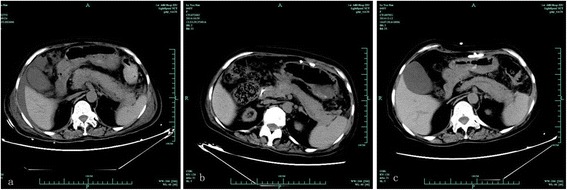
image of CT scan during the the whole course of AP. a Twentie-five days post transplantation, the CT scan of abdomen showed pancreatic swelling, peripheral exudate effusion, considered acute pancreatitis (grade D on Balthazar score, no necrosis visible without contrast injection) after tigecycline treatment for 20 days. b scan showed a basically normal after tigecycline discontinuation for 14 days. c CT reported a normal finding after tigecycline discontinuation for 58 days
